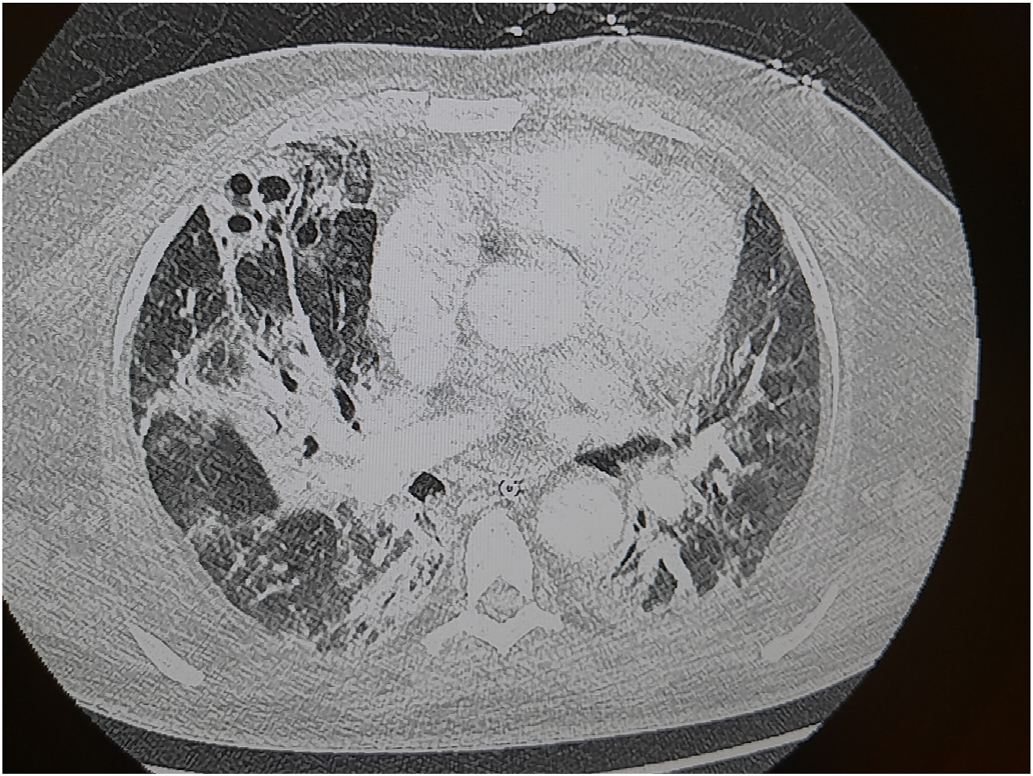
Chest computed tomography-scan performed on day 31 after onset of symptoms showed ground glass opacities and condensations with excavations in right middle lobe.
